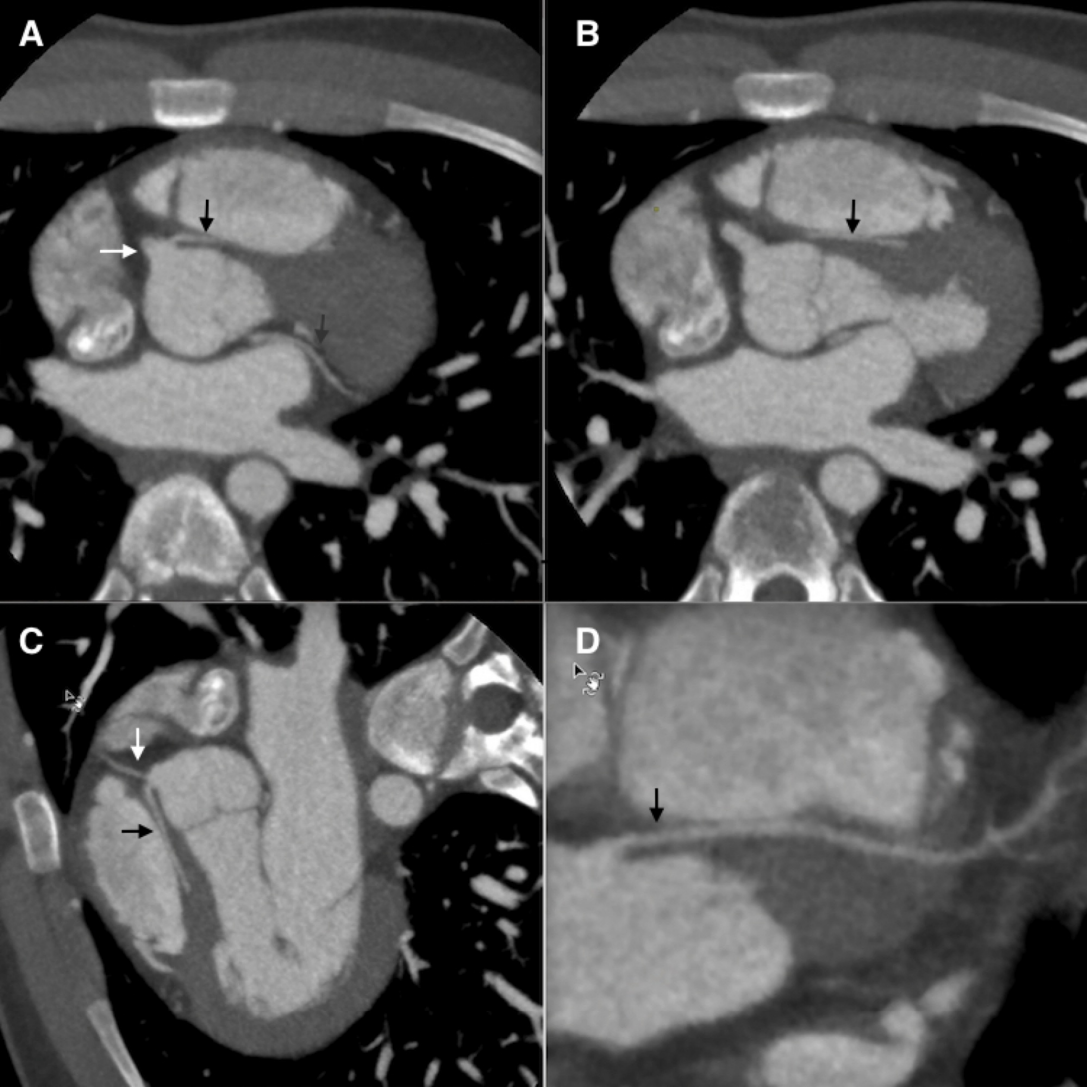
(A) Axial image obtained by 64-slice multidetector computed tomography (MDCT) showing origin of large dominant right coronary artery (white arrow) and anomalous origin of small left anterior descending artery (black arrow) from the right coronary sinus. Small left circumflex artery (gray arrow) is also seen in the image. No plaque was seen in any of three vessels. (B) Anomalous left anterior descending artery (black arrow) seen coursing between ascending aorta and pulmonary trunk. (C) MDCT maximal intensity projection demonstrating anomalous origin and malignant course of left anterior descending artery (black arrow). Also notice origin of small left circumflex artery (white arrow) from right coronary sinus. (D) MDCT image demonstrating a multiplanar reconstruction of the entire anomalous left anterior descending artery (black arrow).
